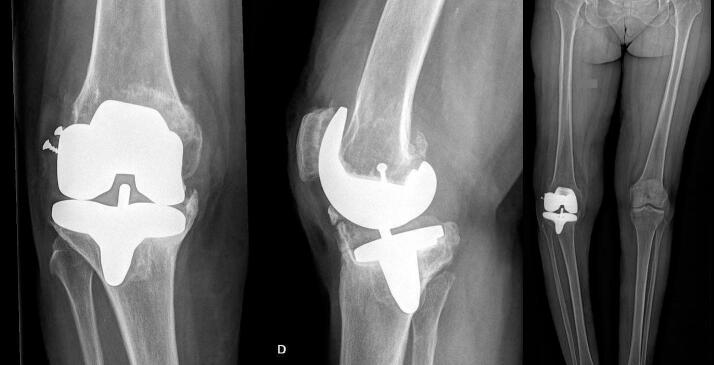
Right knee preoperative (a) anteroposterior; (b) lateral; (c) long leg view radiographs. These are demonstrating massive prosthetic loosening and migration of the components with severe right knee varus (HKA of 162°).
